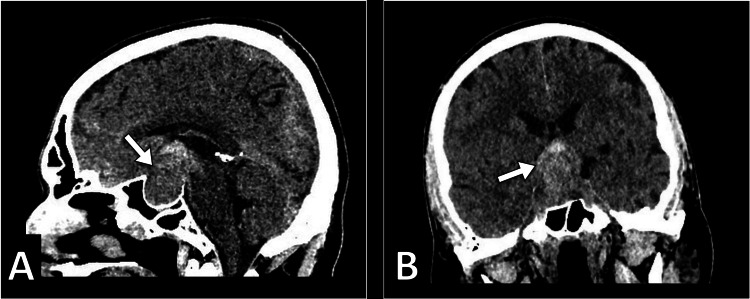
Sagittal (A) and coronal (B) CT images show a large intrasellar mass (arrow) with areas of high density suggestive of hemorrhage in the pituitary gland CT: computed tomography
